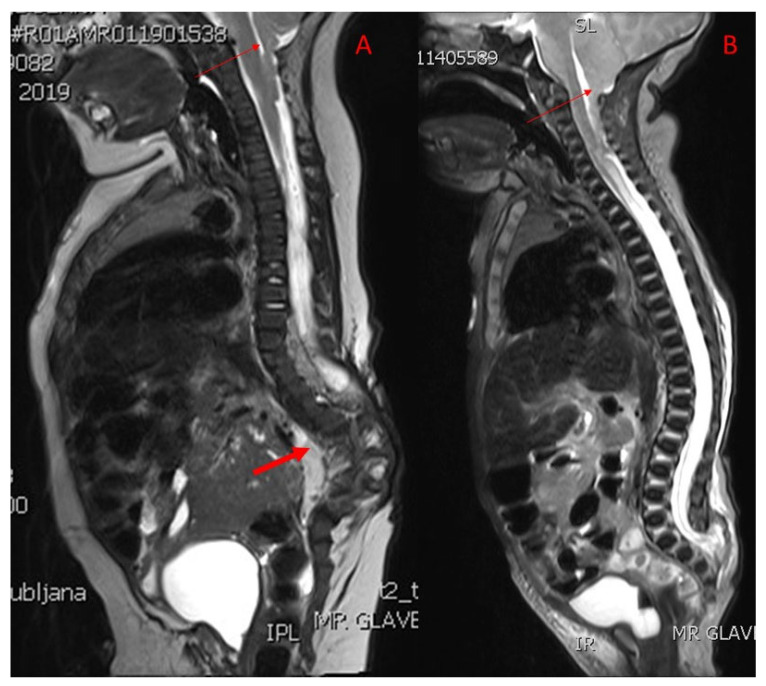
A T2- weighted MRI sagittal image of spine, showing (A) extreme lumbar kyphosis (thick arrow) and Chiari II malformation (thin arrow) and (B) Chiari II malformation (thin arrow) with pronounced syringobulbia in a previously surgically treated children.
